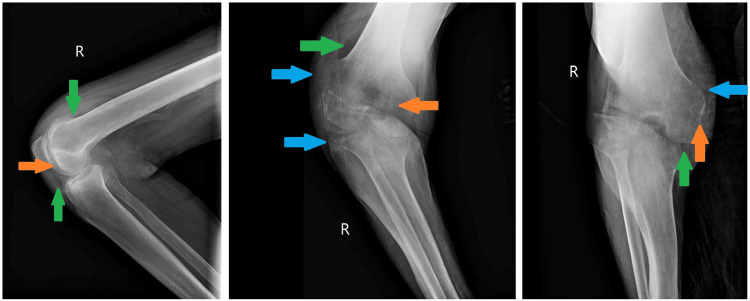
Three-view X-ray of right knee showing flexion deformity, mild degenerative changes (orange arrows), joint effusion (green arrows), and soft tissue swelling (blue arrows)
